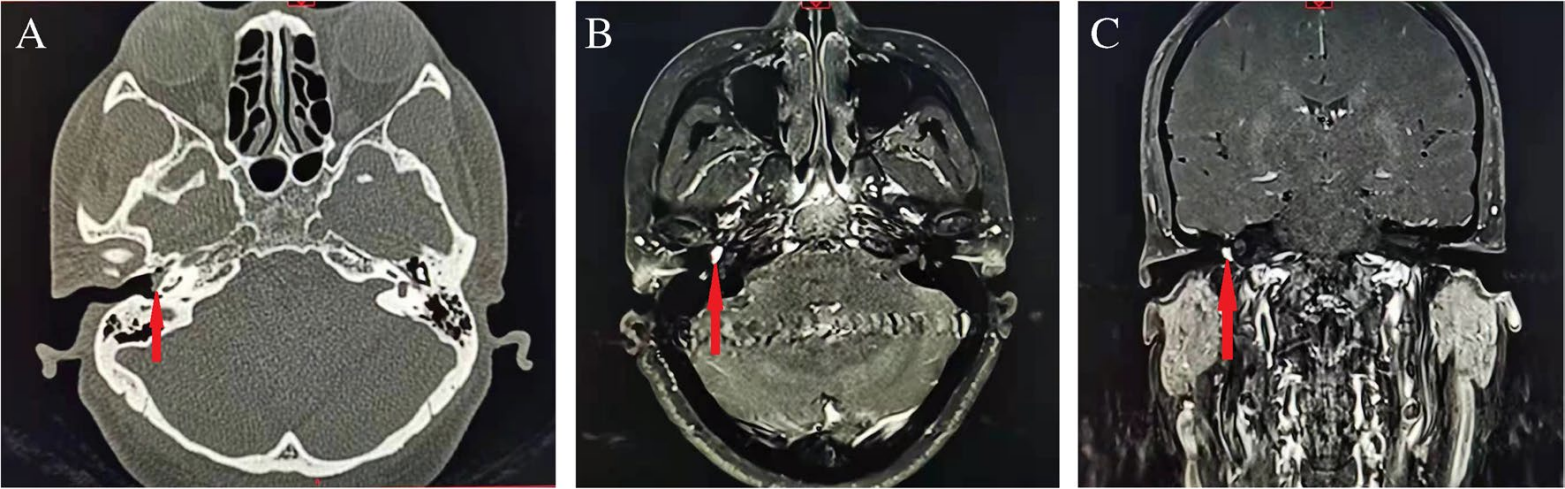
A tympanomastoid paraganglioma in the right tympanic cavity. A Axial CT scan. B, C Axial (B) and coronal (C) T1-weighted contrast-enhanced MRI. Arrows indicate a mass in the right middle ear lateral to the cochlear promontory.
